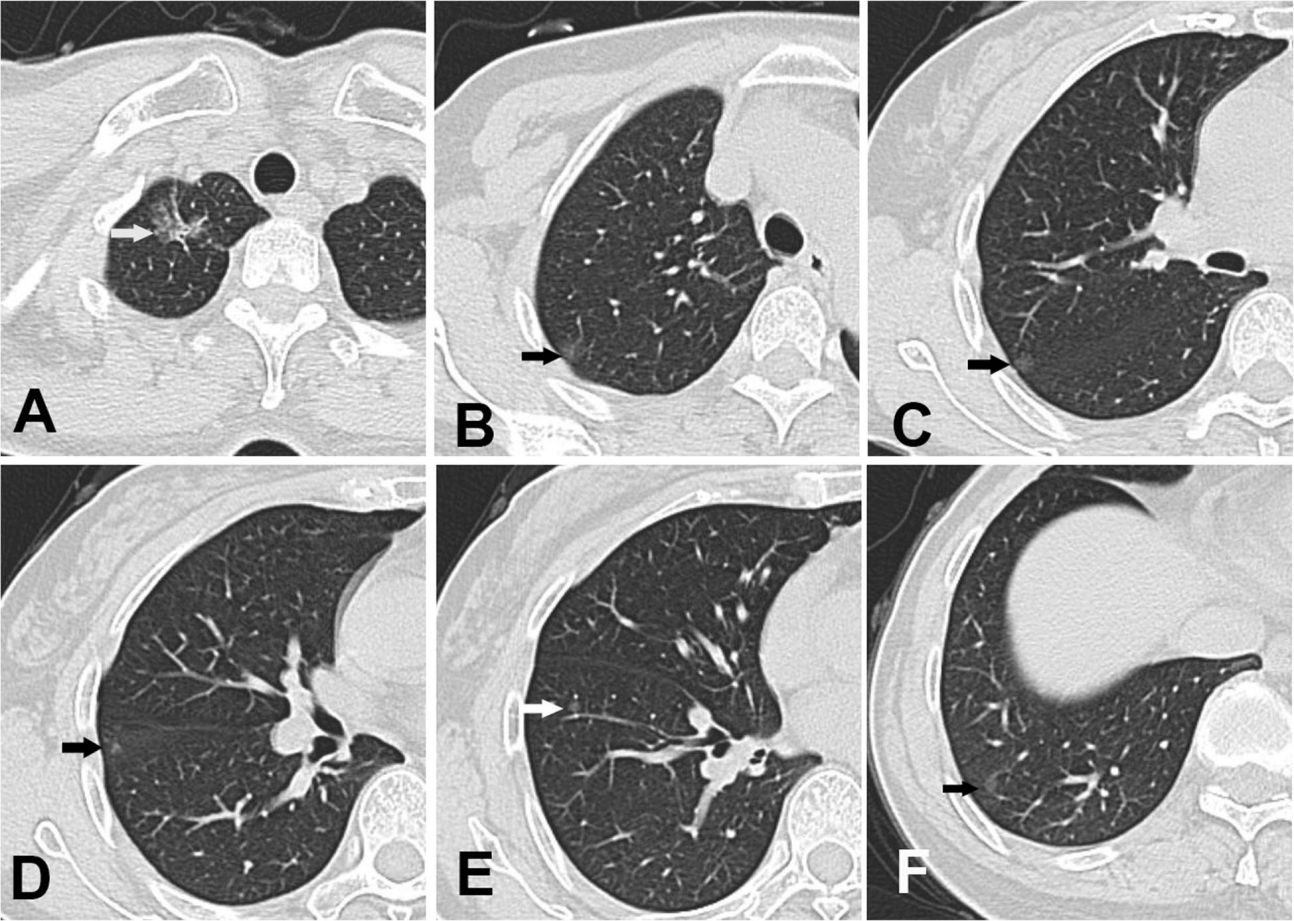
High-resolution computed tomography images of patient 1 reveal six ground-glass opacity (GGO) lesions in the right lobe (arrows). a One nodule in the apical segment of the right upper lobe. b-d Three nodules in the posterior segment of the right upper lobe. e, f Two lesions in the right lower lobe
